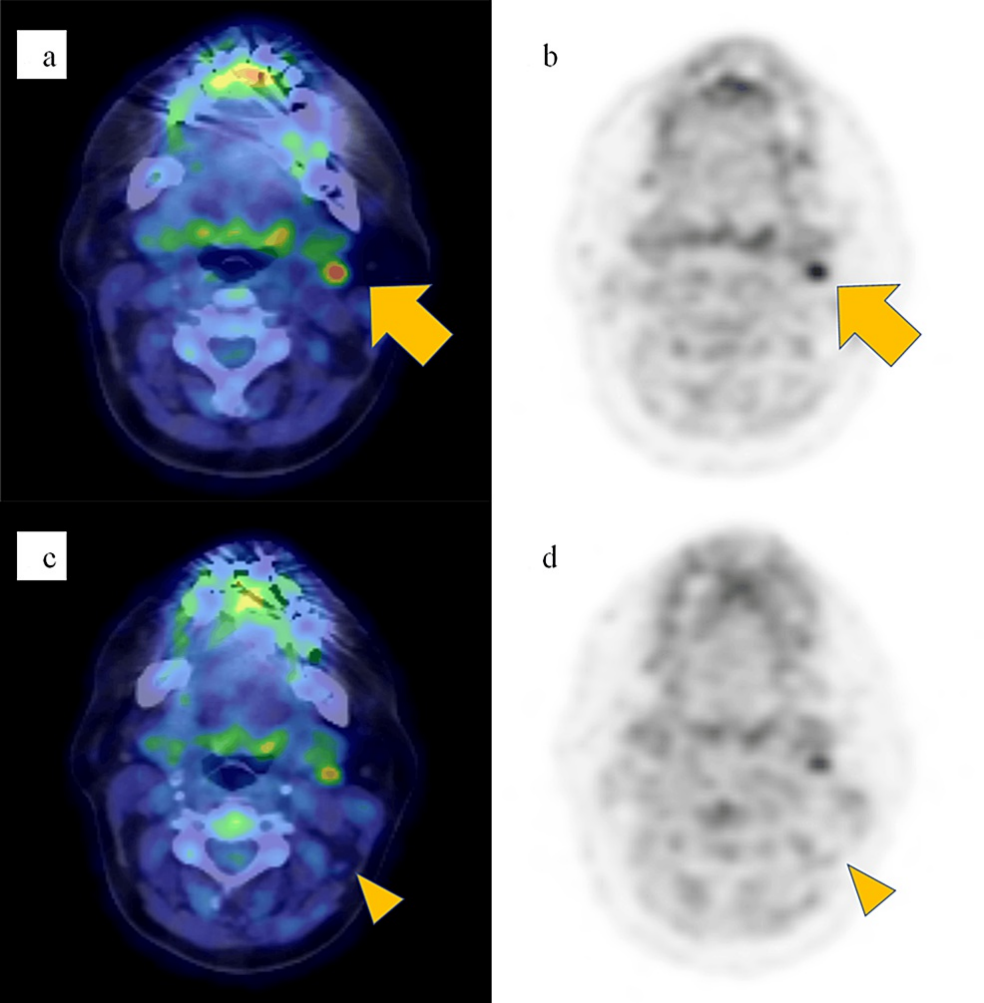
Radiological findings about four months after the start of chemotherapy. (a, b) An ^18^F-fluorodeoxyglucose-position emission tomography (FDG-PET) image in the delayed phase/computed tomography, a fusion image acquired in the axial plane, and an FDG-PET image in the delayed phase acquired in the axial plane show reductions in size and FDG accumulation (standardized uptake value max of 3.8 in the early phase, and 5.2 in the delayed phase) compared with the initial examination at a cervical lymph node (arrow). (c, d) An FDG-PET image in the delayed phase/computed tomography, a fusion image acquired in the axial plane, and an FDG-PET image in the delayed phase acquired in the axial plane show reductions in the size of a lymph node with almost complete disappearance of FDG accumulation (arrowhead).
